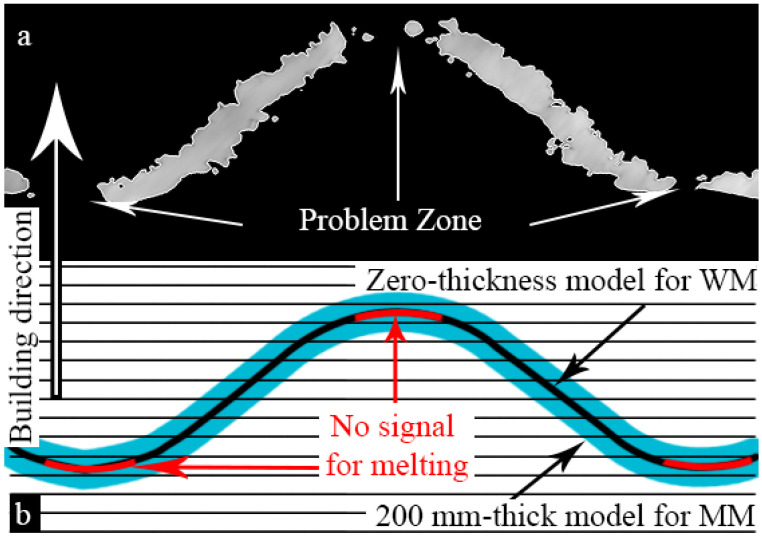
Assumption on the through-holes’ appearance: (a) CT in vertical cross-sectional view; (b) Scheme of manufacturing process in vertical cross-sectional view.
